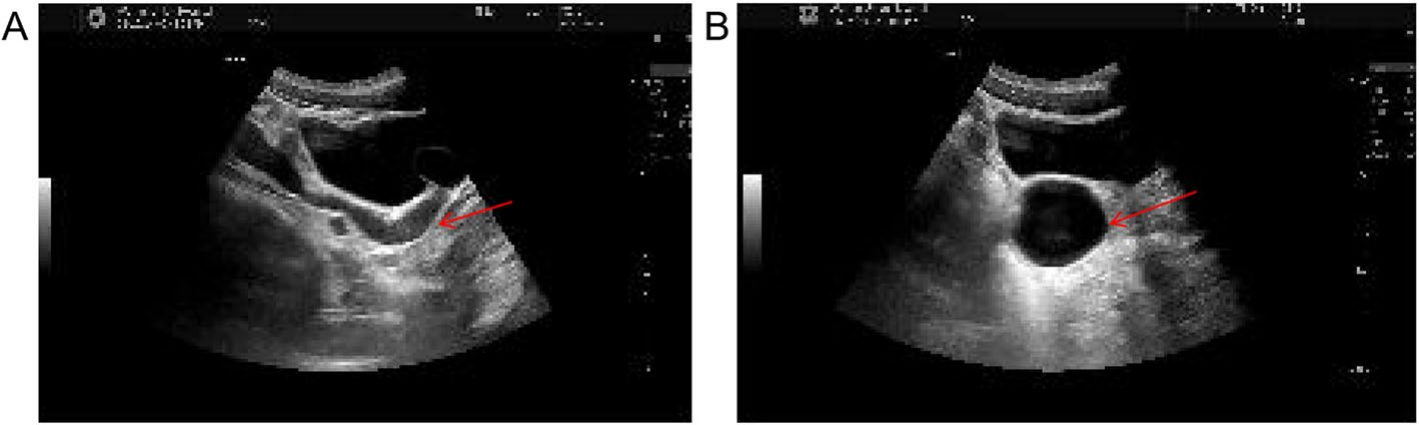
Ultrasonographic findings in the patient. A An anechoic region indicative of an abnormality was shown along the mid-lower ureteral pathway, as highlighted by the red arrow. B A cystic anechoic area measuring 57.5 × 44.5 mm was presented near the left seminal vesicle gland, also marked by a red arrow
